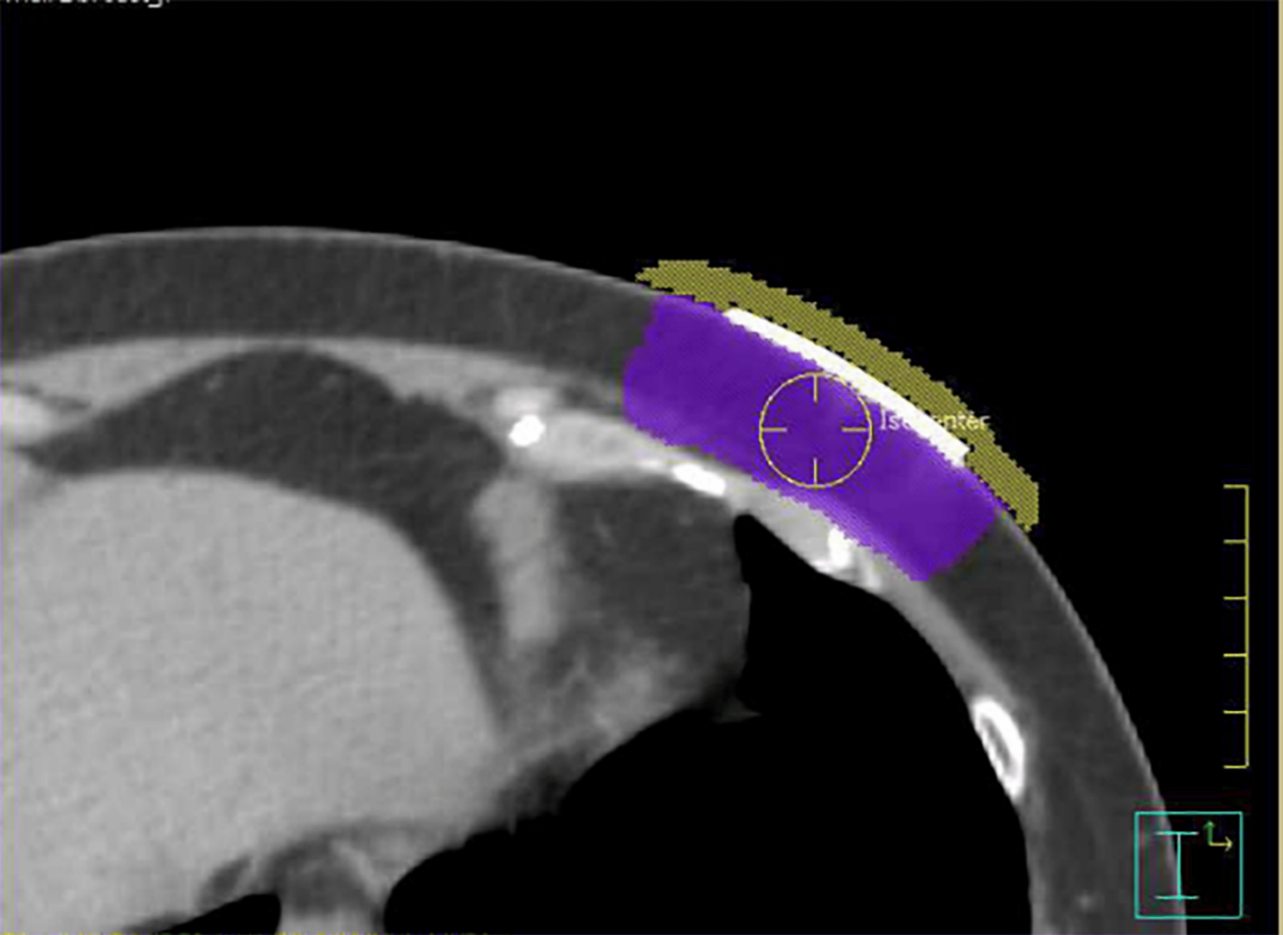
Axial CT slice showing bolus (yellow) and PTV in the left chest wall (purple). PTV: planning target volume
